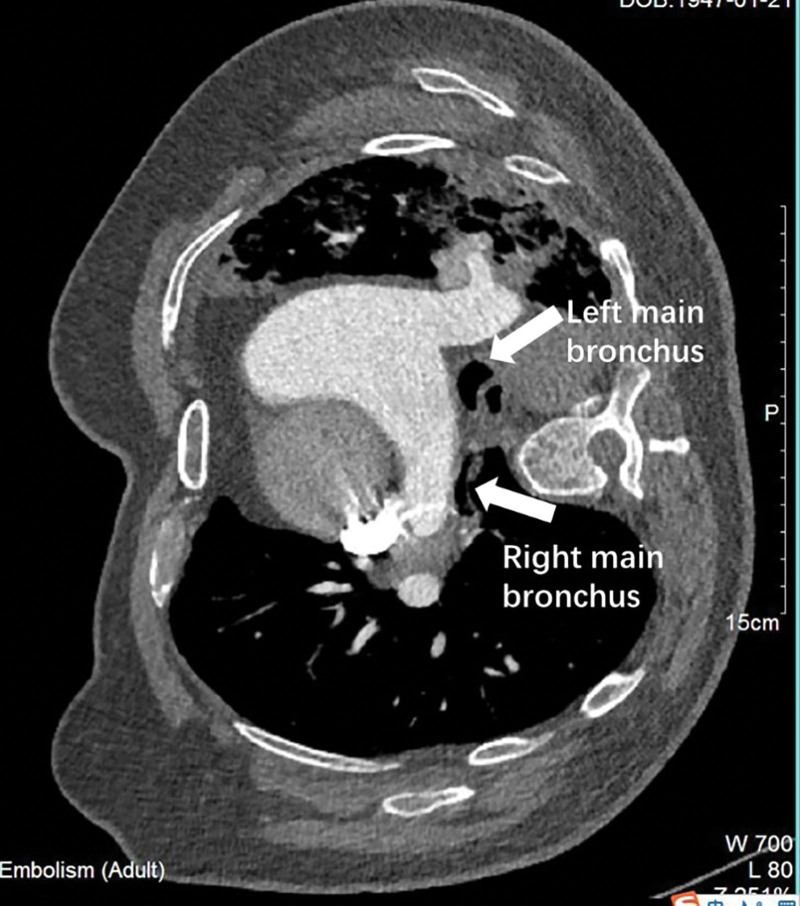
CT scan: The collapse of right main bronchus during an expiratory phase CT in right recumbent position. CT = computed tomography.
